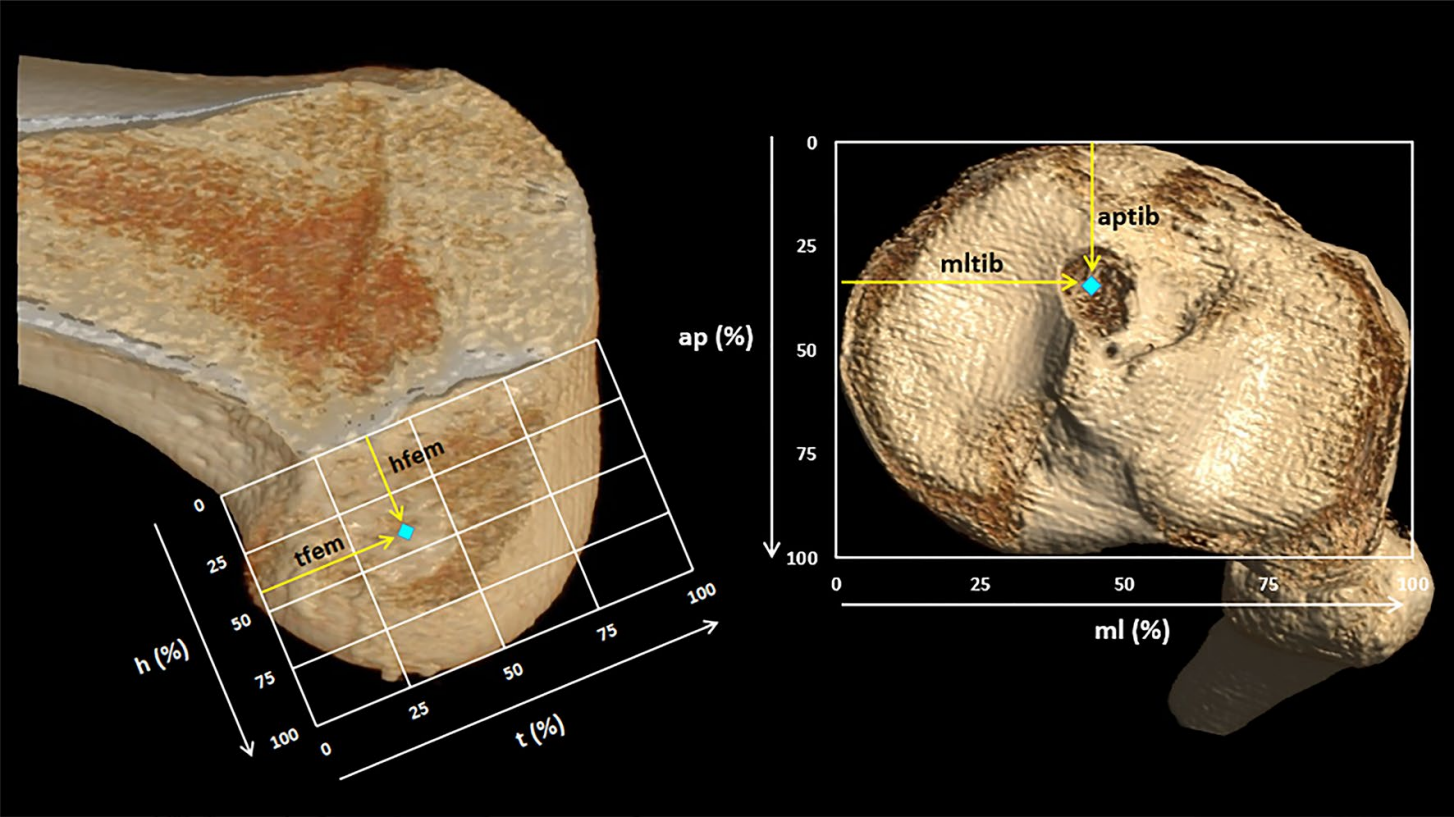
3D-CT view of the medial side of the lateral femoral condyle with the quadrants of Bernard (left) and the tibial plateau (right). Blue dot: Example tunnel aperture position. Femur: h: the total superior-inferior height of the quadrants, starting at 0% perpendicular at the Blumensaat line and ending at 100% at the end of the femoral condyle. t: the total posterior-to-anterior length of the quadrants, starting at 0% at the most posterior part of the condyle, parallel to Blumensaat and ending at 100% at the most anterior part. tfem: posterior-to-inferior position of the tunnel aperture, expressed in a percentage of t. hfem: superior-inferior position of the tunnel aperture, expressed in a percentage of h. Tibia: ap: the total anterior-to-posterior length of the quadrants, starting at 0% at the most anterior part of the tibial plateau and ending at 100% at the most posterior part of the tibial plateau. ml: the total medial-to-lateral length of the quadrants, starting at 0% at the most medial part of the tibial plateau and ending at 100% at the most lateral part of the tibial plateau. aptib: anterior-to-posterior position of the tunnel aperture, expressed in a percentage of ap. mltib: medial-to-lateral position of the tunnel aperture, expressed in a percentage of ml
